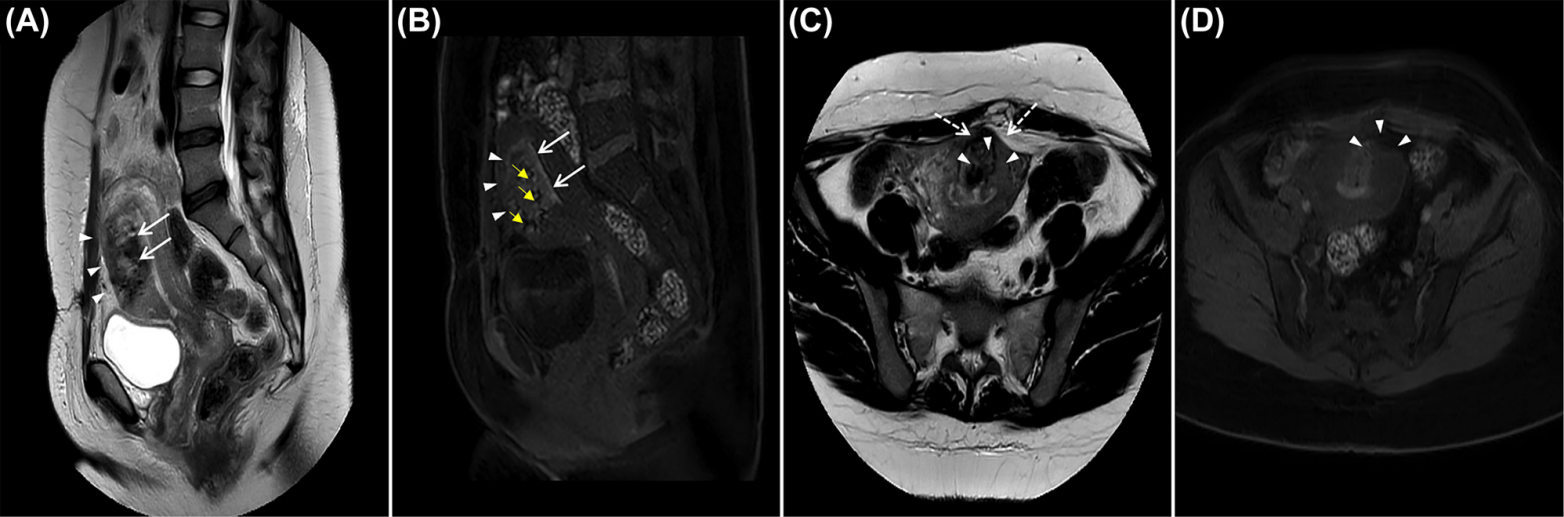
Sagittal and axial T2 MRI images (A, C) obtained on post-operative day 20. The images demonstrate a large, relatively ill-defined hypointense area involving almost the entire anterior myometrium in the midline with a sharp line of demarcation posteriorly at the anterior junctional zone (white arrows). This corresponds to sagittal and axial precontrast T1 MRI images (B, D) from the same scan that show an ill-defined infiltrating hyperintensity in the same area (white arrow heads). A dark “hemosiderin ring” is noted on T2 (white dashed arrows). Susceptibility artifacts (yellow arrows B) indicate the hysterotomy site.
